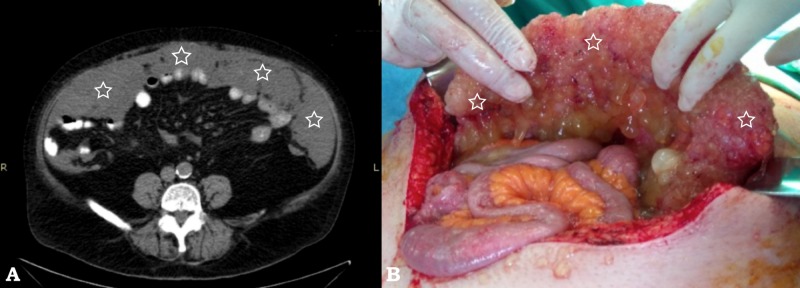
Pseudomyxoma peritonei, (patient #1) (A) Axial computed tomography (CT) slice demonstrates a large hypodense lobulated omental mass (white stars) and (B) demonstrates a corresponding perioperative view of the greater omentum with extensive tumor seeding (white stars)
